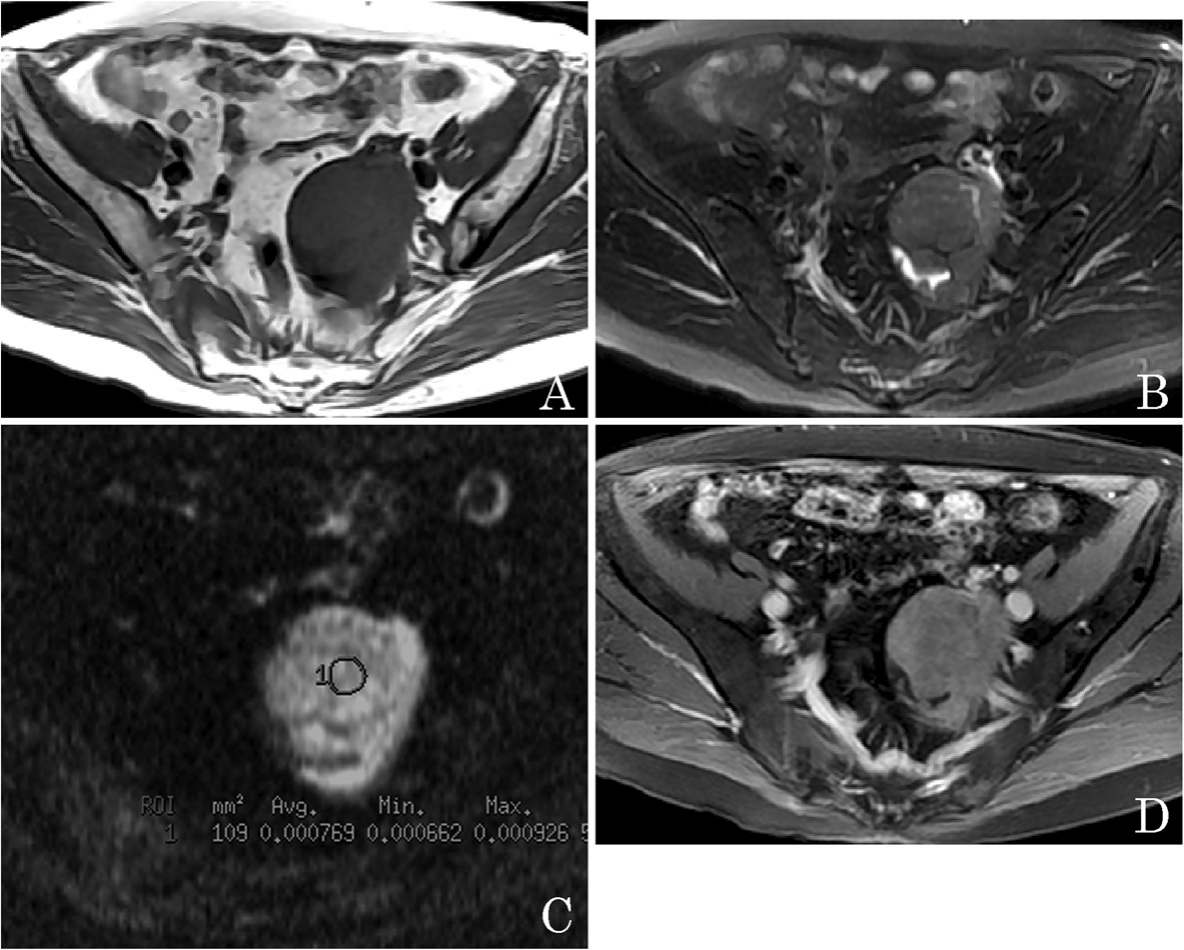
A 63-year-old woman with a left ovarian cystadenocarcinoma. (A) An axial T1-weighted image shows a left pelvic mass with isointensity. (B) Axial T2-weighted image reveals that the mass is a solid tumor with low signal intensity. (C) Axial diffusion-weighted imaging (DWI) obtained at b = 1,000 s/mm^2^ reveals the solid tumor as hyperintense with a lower apparent diffusion coefficient (ADC) value (circle 1, ADC = 0.769 × 10^-3^ mm^2^/s). (D) Axial contrast-enhanced T1-weighted image demonstrates marked enhancement of the solid part.
